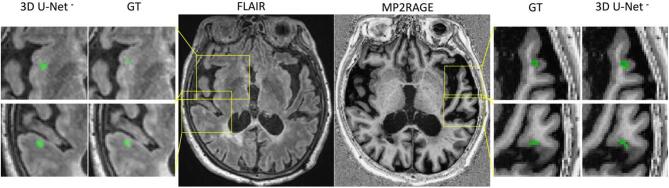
Four examples of correctly detected cortical lesions by our proposed method. All lesions are better visible in the MP2RAGE sequence compared to the FLAIR.
